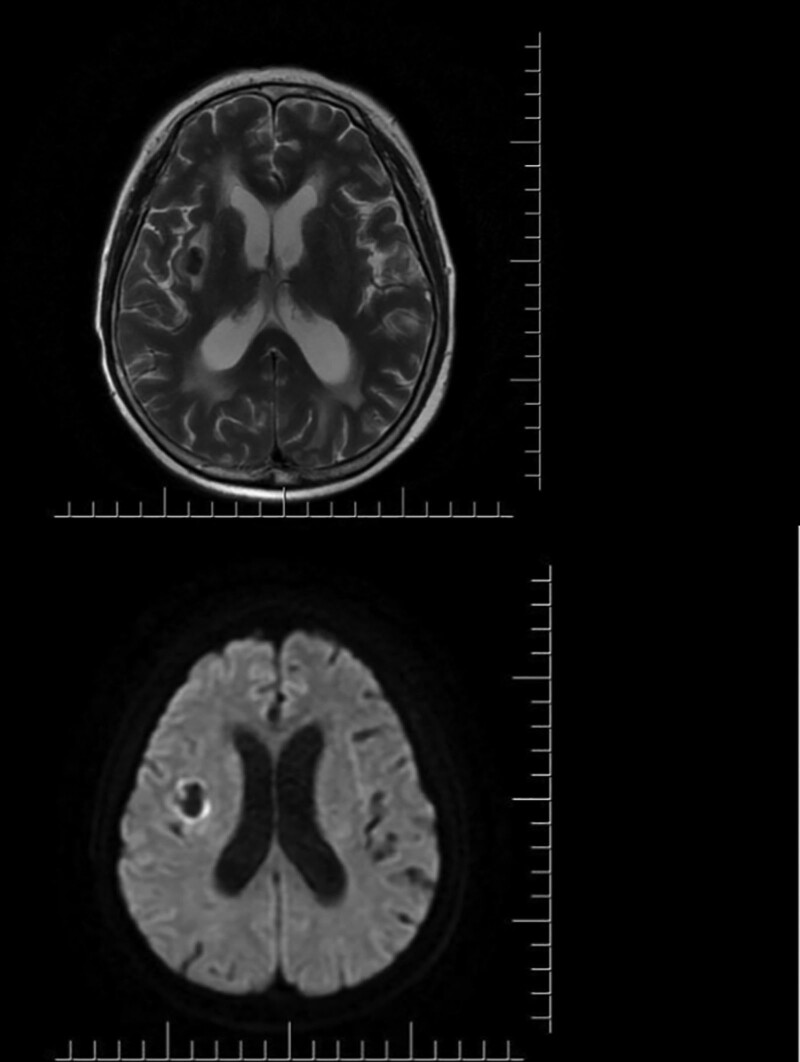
MRI: T2: low signal in the right outer sac, DWI: annular hypersignal in the right outer sac. DWI = diffusion-weighted imaging, MRI = magnetic resonance imaging.
